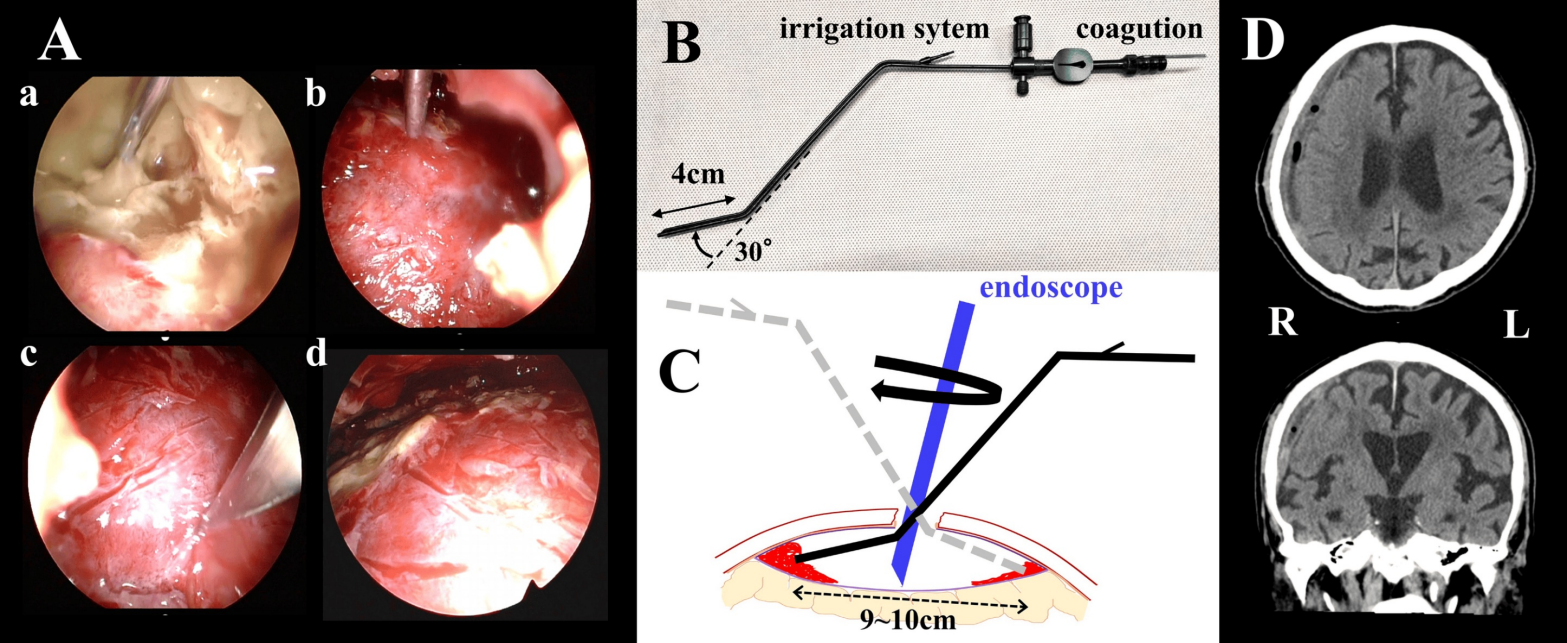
Endoscopic surgery and the result Aa: Jelly-like white clots were aspirated by suction as much as possible; b: the hard inner membrane was exposed, no brain pulsations were recognized due to this hard membrane; c: incisions were made gently with a sharp blade, without damaging the deeper inner membrane or stiffened pia mater on the brain surface; d: when multiple incisions had been made in the inner membrane, simultaneous brain expansion and pulsation were observed. B: The suction device used to remove clots deep in the hematoma cavity was fabricated by the author in an original shape, with a 30°bend at the 4 cm tip; the device possesses irrigation and coagulation capabilities. Figure 2B is created by the author, Shoko M. Yamada. C: The 4 cm tip can be inserted simultaneously with the endoscope into the hematoma cavity without damaging brain tissue and can be rotated 180° to the left or right to allow irrigation and suction of the entire circumference of the cavity. It is effective for a cavity of 9–10 cm in diameter, including the diameter of the burr-hole. Figure 2C is created by the author, Shoko M. Yamada. D: CT performed three days after surgery, showing definite brain expansion.
